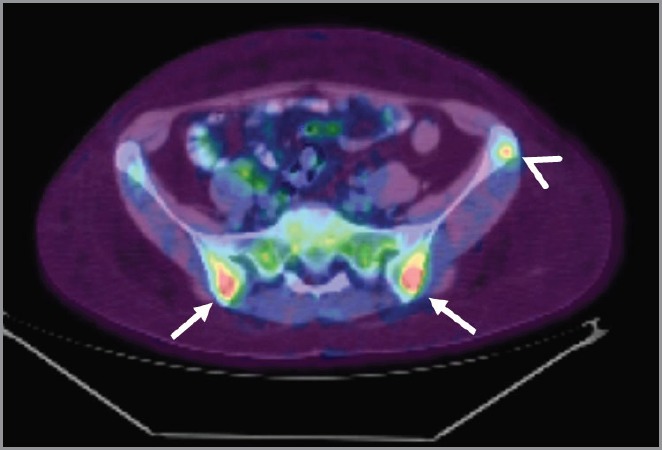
Pelvic bone lesions on ^18^F-FDG-PET/CT. ^18^F-FDG-PET/CT showed ill-defined lesions in the iliac bones (arrow) and a round-shaped lesion in the left iliac crest (arrowhead) with increased ^18^F-FDG-uptake.
